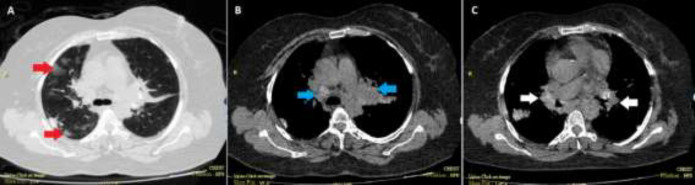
The chest high resolution computed tomography of the patients. A) The red arrows show multiple nodules in the upper lobe of right lung. B) In the aortopulmonary window, paratracheal lymphadenopathies are shown by blue arrows. C) Hilar lymphadenopathies are shown by white arrows
